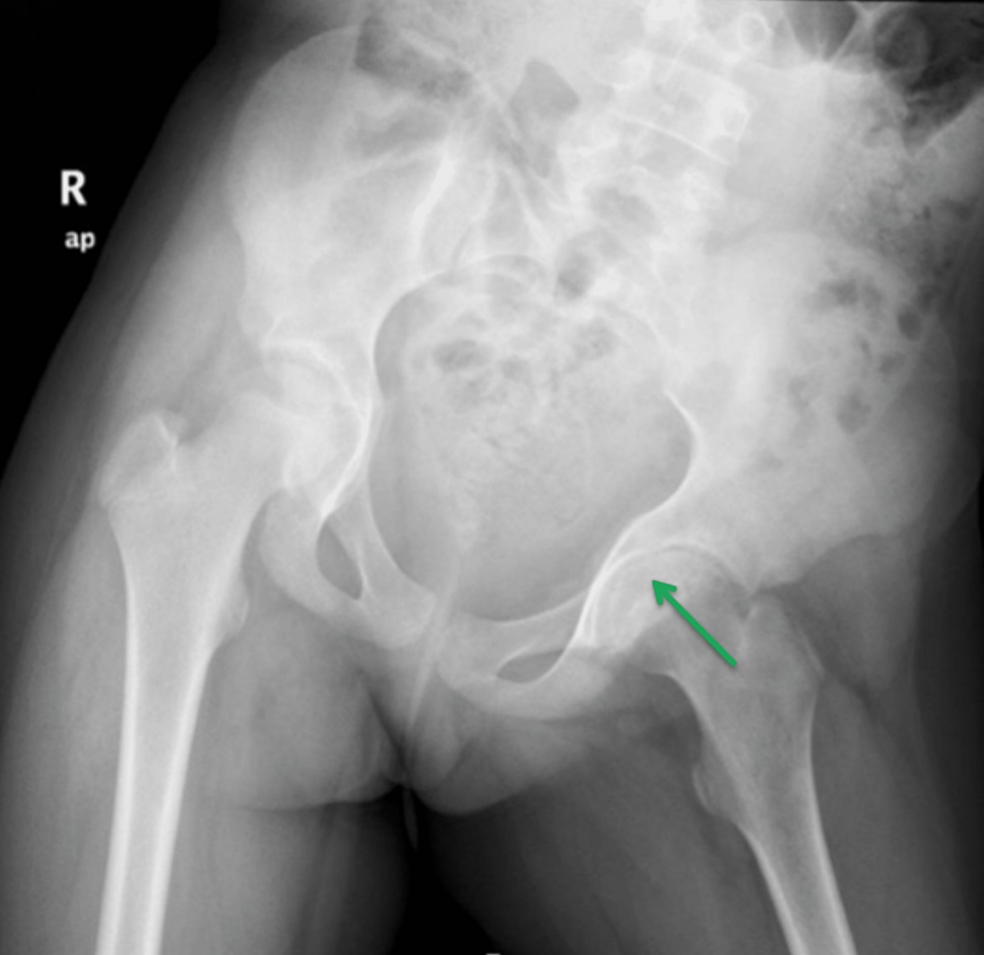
Initial anteroposterior pelvis radiograph showing concentric narrowing of the left hip joint space and reduced bone density (green arrow). Pelvic tilt to the left side can be seen.
